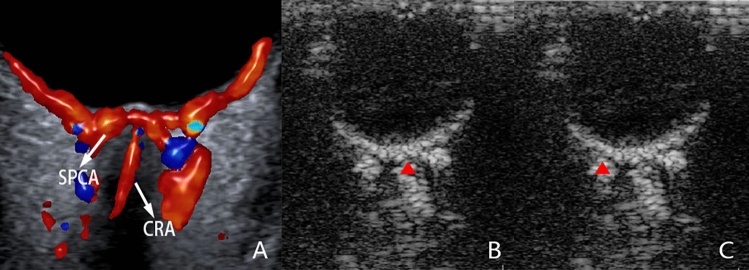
A The position of CRA and SPCA behind the eyeball in color Doppler mode (white arrow). B The triangle is the region of interest selected for CEUS quantitative analysis of the CRA. C The triangle is the region of interest selected for CEUS quantitative analysis of the SPCA. Abbreviations: *CEUS* contrast-enhanced ultrasound, *CRA* central retinal artery, *SPCA* short posterior ciliary artery
